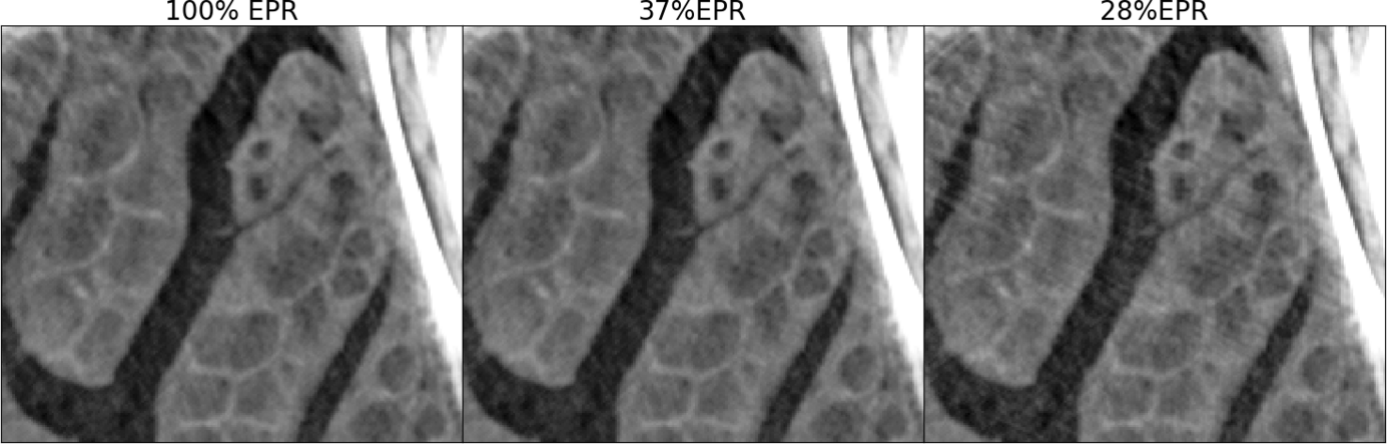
Hybrid scheme results, where only a fraction of each radiography is processed with EPR and the remaining part with Paganin, according to automatic detection of the critical regions. From left to right the results are shown for an overall average of 100%, 37% and 27% of the total projection volume processed with EPR. For an EPR coverage of 37% the difference with the 100% case is barely visible.
